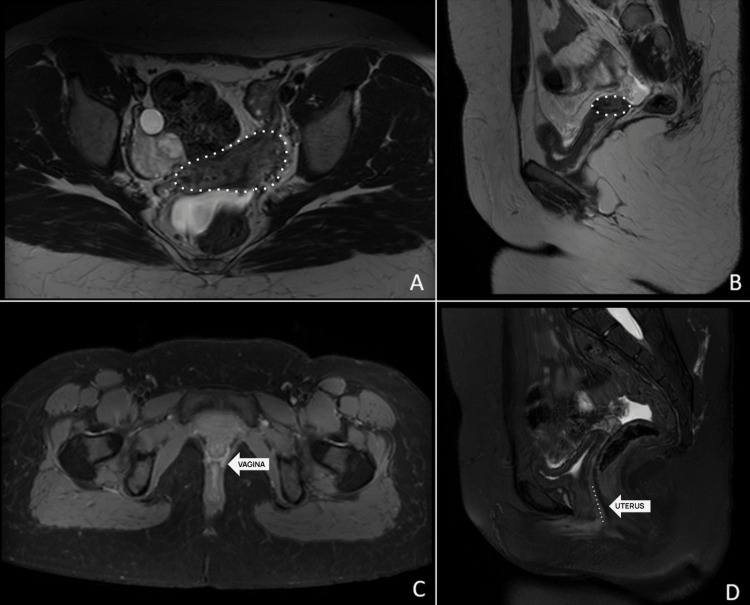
Pelvic Magnetic Resonance Imaging (A) Axial T2, (B) Sagittal T2, (C) Axial T1 with Weighted Image and Contrast, and (D) Sagittal T2 Weighted Image (A) A white-outlined area with a hypoplastic uterus is observed, with a small rudimentary uterine structure and no discernible endometrial cavity. (B) A white-circumscribed area confirms the presence of a small, underdeveloped uterine remnant, lacking an endometrial stripe and distinct uterine layers. The uterine body appears hypointense, without normal anatomical landmarks. (C) The arrow points to the vagina, identified as the H-shaped hyperintense structure. It appears small, with poor contrast enhancement, indicating underdevelopment (D) The arrow points to a white straight line that reflects the path of the hypoplastic uterus located posterior to the urethra. This line clearly delineates the hypoplastic uterine remnant from the surrounding pelvic fat, with no evidence of functional endometrial tissue in the underdeveloped uterine structure.
